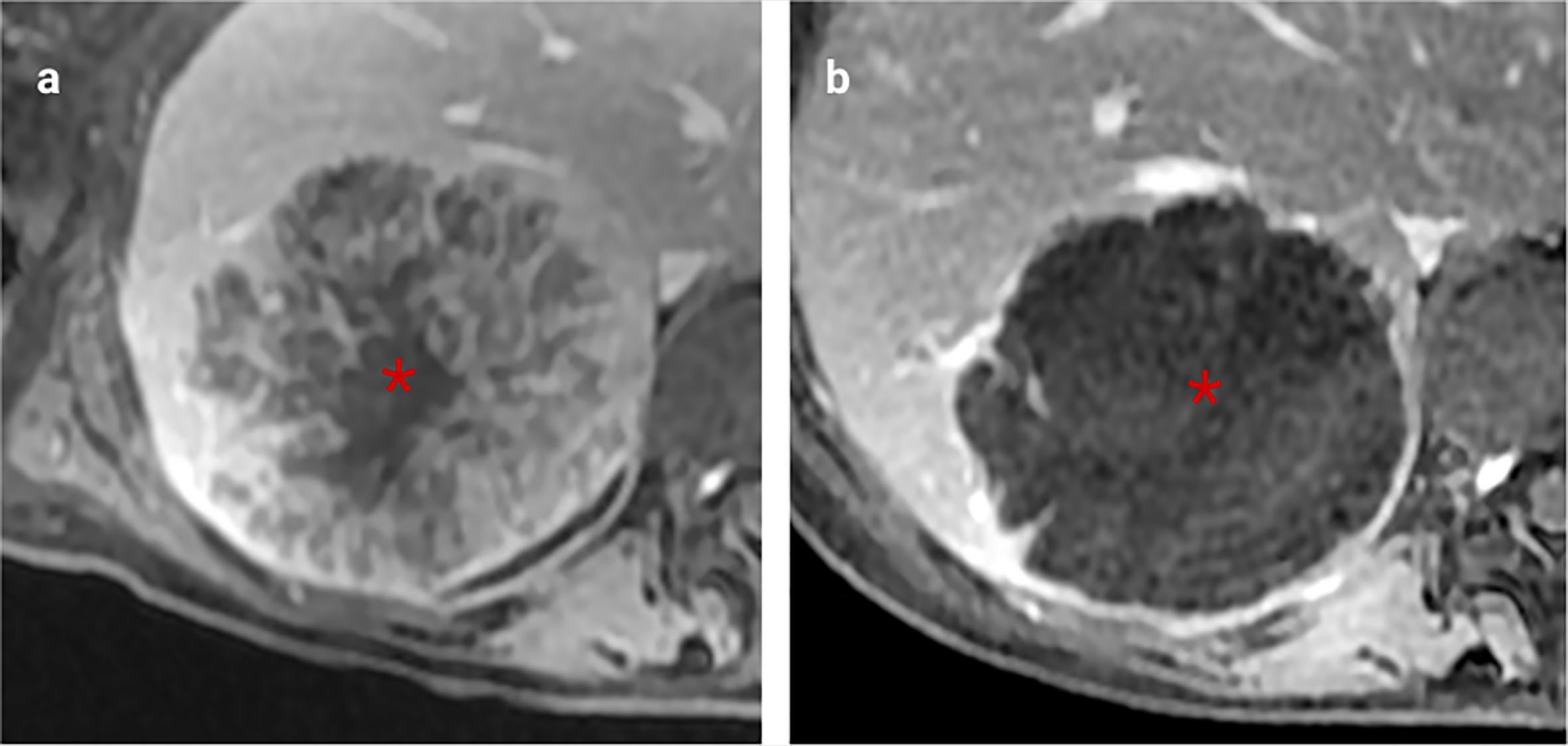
Excellent morphological response to chemotherapy. (A). Heterogenously enhancing CRLM on portal venous phase T1-weighted MRI (asterisk). (B), Post-chemotherapy MRI shows the lesion has become homogenous without a reduction in size, indicating a significant decrease in viable tumour cells.
